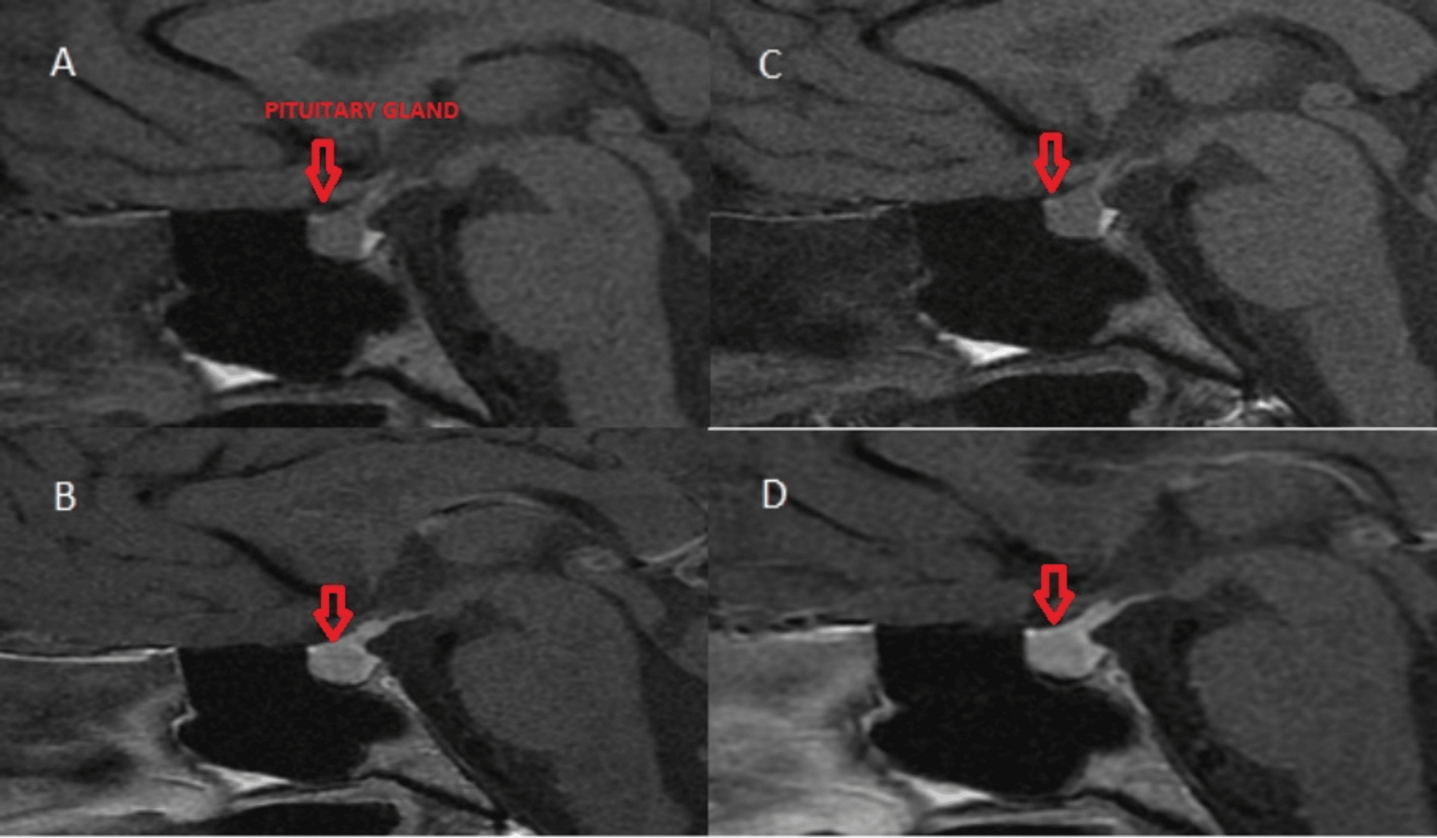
Control MRI of the hypothalamic-pituitary region in the sagittal plane shows no changes in the findings A: native series, B: contrast series (22 months after the first MRI), C: native series, D: contrast series (31 months after the first MRI) MRI: magnetic resonance imaging
